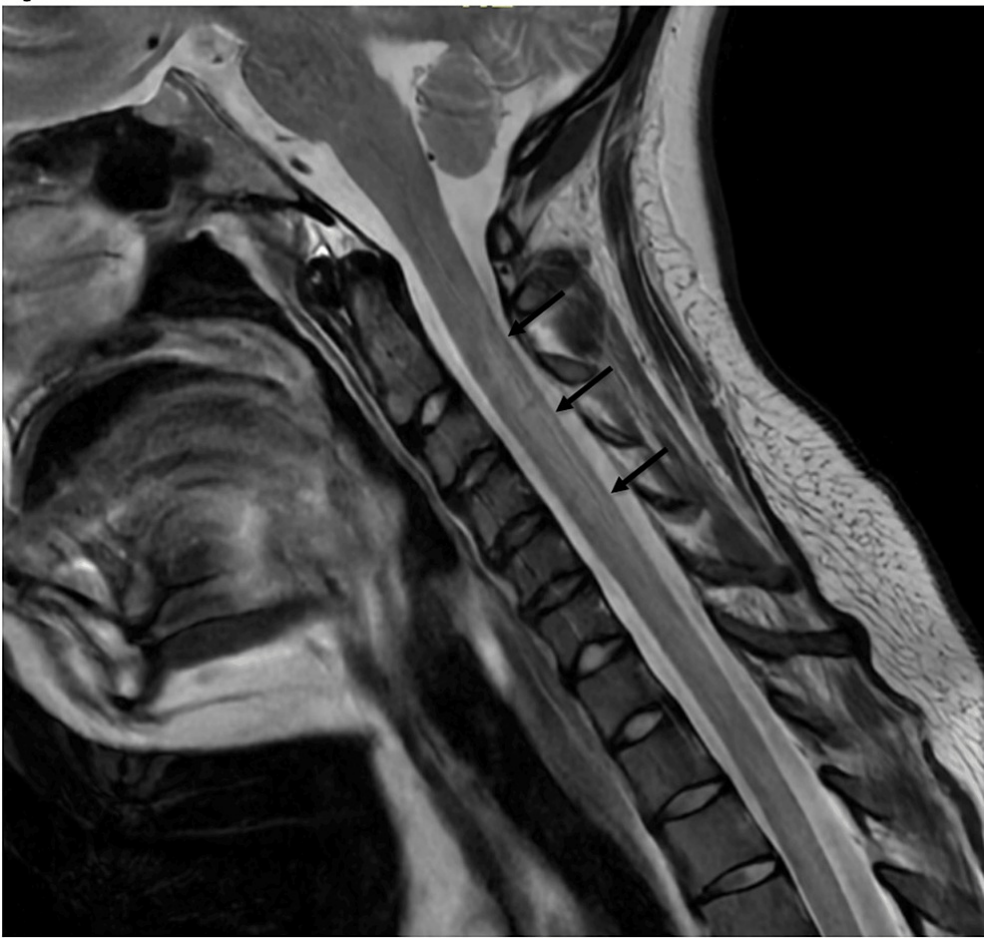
Laughing gas-induced subacute combined generation from nitrous oxide. MRI of the cervical and thoracic spine with and without contrast revealed a T2 hyperintensity (arrows) within the dorsal columns of the spinal cord, most severe throughout the upper cervical spinal cord extending from C2 through C5-C6.
